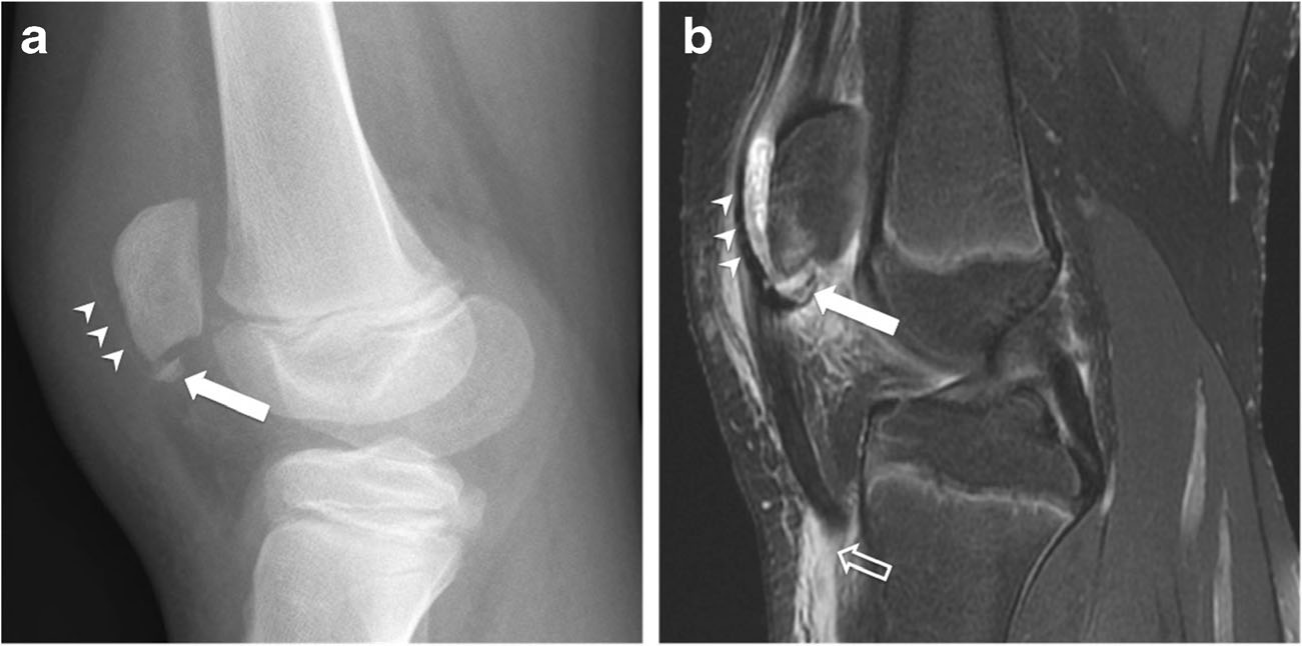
Sleeve fracture of the lower patellar pole in an 11-year-old boy after falling on his flexed knee during a football game, with a popping sound. The patient presented to the ER 5 days later. (a) Lateral knee radiograph and (b) sagittal fat-suppressed T2-weighted MR image 3 days after knee x-ray show small bony fragment fracture from the lower pole (*arrow*), extending anteriorly, with detachment of a thin shell of cortical bone (*arrowheads*), consistent with a sleeve fracture. Note the injury of the distal attachment of the patellar tendon (*open arrow*). *Images courtesy of Arnold Carlson Merrow, Jr., MD - Cincinnati Children’s Hospital Medical Center, OH, USA*
